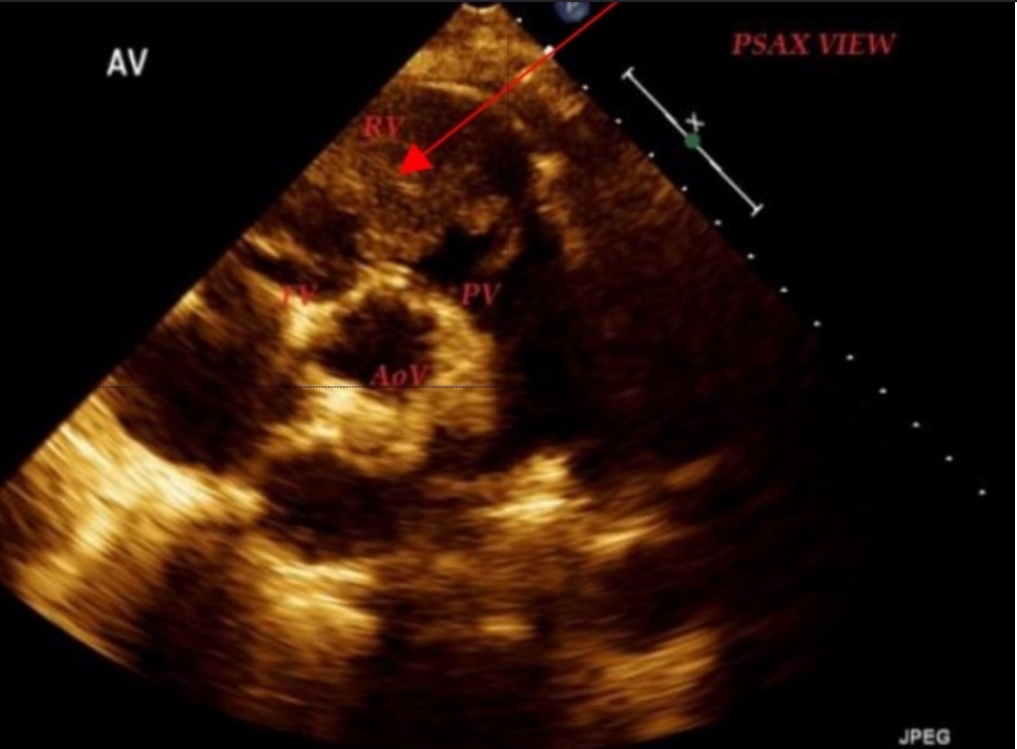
Parasternal short axis view echocardiographic image showing a large fungating mass in the right ventricle RV: right ventricle; PV: pulmonary valve; AoV: aortic valve; TV: tricuspid valve.
